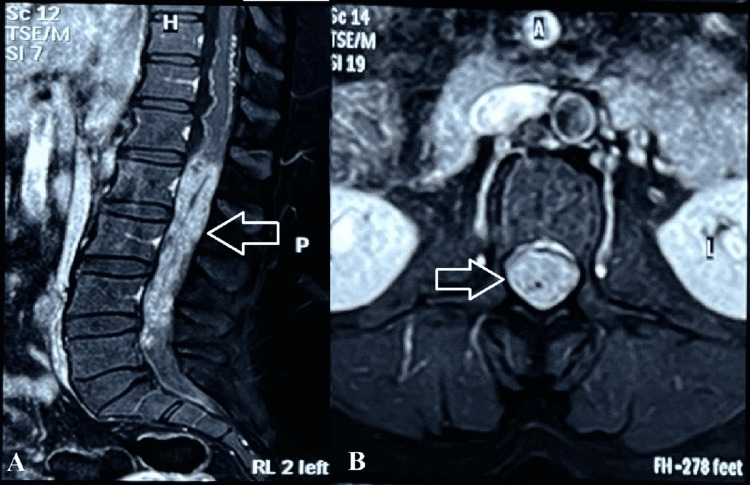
Post-gadolinium contrast T1-weighted MRI sequences show intradural enhancement extending from the L1 to L5 levels in the sagittal (A) and at the L2 level in the axial (B) cuts.
